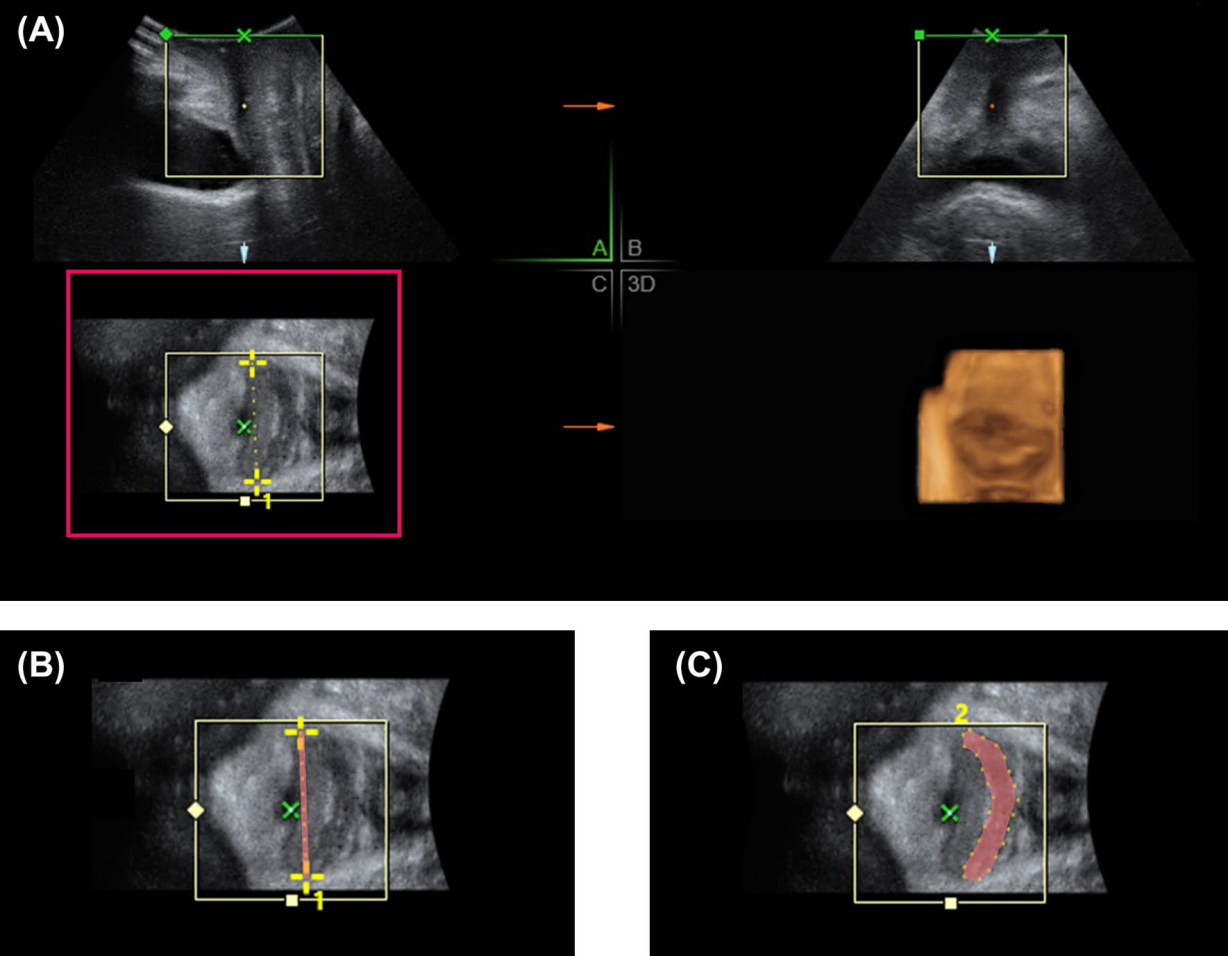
The measurement of vaginal topography via transperineal ultrasound. A convex probe was placed over perineum. The measurement was done on the midsagittal plane. Mode of rendered volume was obtained on relax without pelvic muscle contraction to obtained an axial plane of pelvis over the panel (C) in (A). The vagina was recognized as hypoechoic area between urethra and anal canal in genital hiatus. Vaginal width (the red line in B) and cross-sectional area of vagina (the red-marked area in C) were measured at level of proximal, middle and distal part of vagina.
